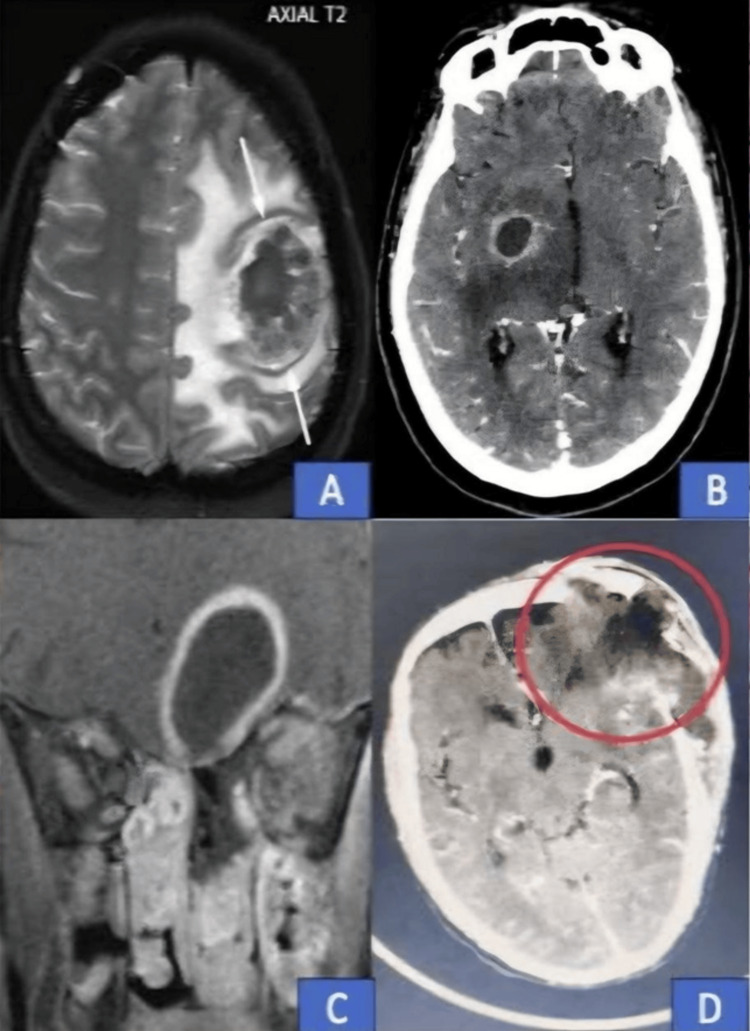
Radioimaging findings. (A) T2-weighted image showing granulomatous inflammation; (B) ring-enhancing lesions in neuromelioidosis; (C) abscess in mucormycosis; (D) infected bone with abscess. (Cases referred to in the text are Cases 4, 8, 10, and 6, respectively.)
